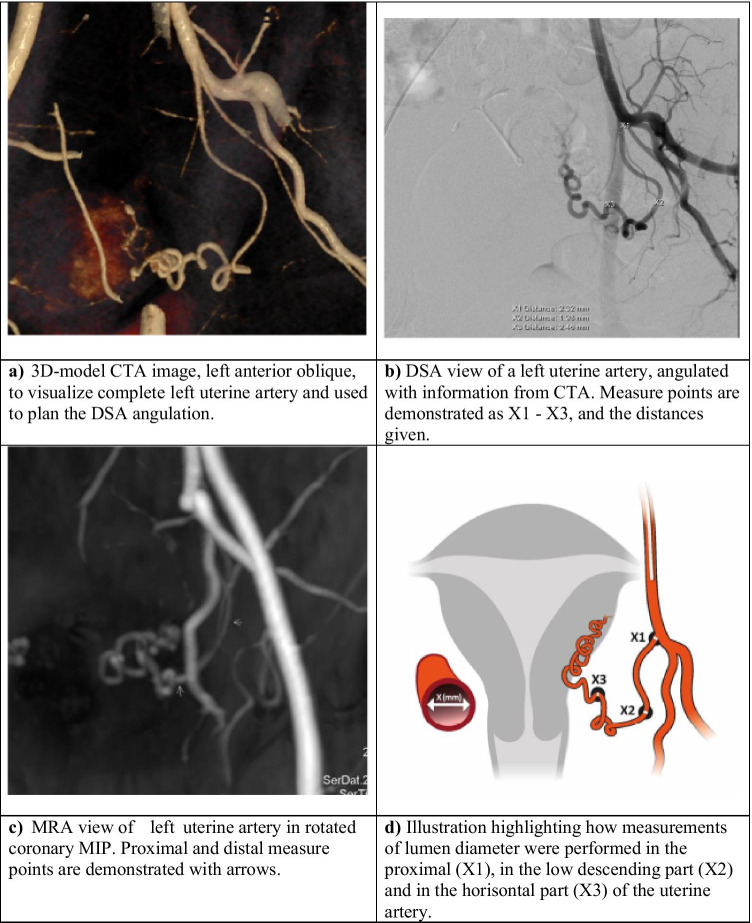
Example of the reproduction of the uterine artery in an oblique plane in the different imaging modalities, (a) CTA, (b) DSA, (c) MRA, and the location of the measurement points are given in d. Abbreviations: CTA = computed tomography angiography, DSA = digital subtraction angiography, MRA = magnetic resonance angiography, and MIP = maximal intensity projection
